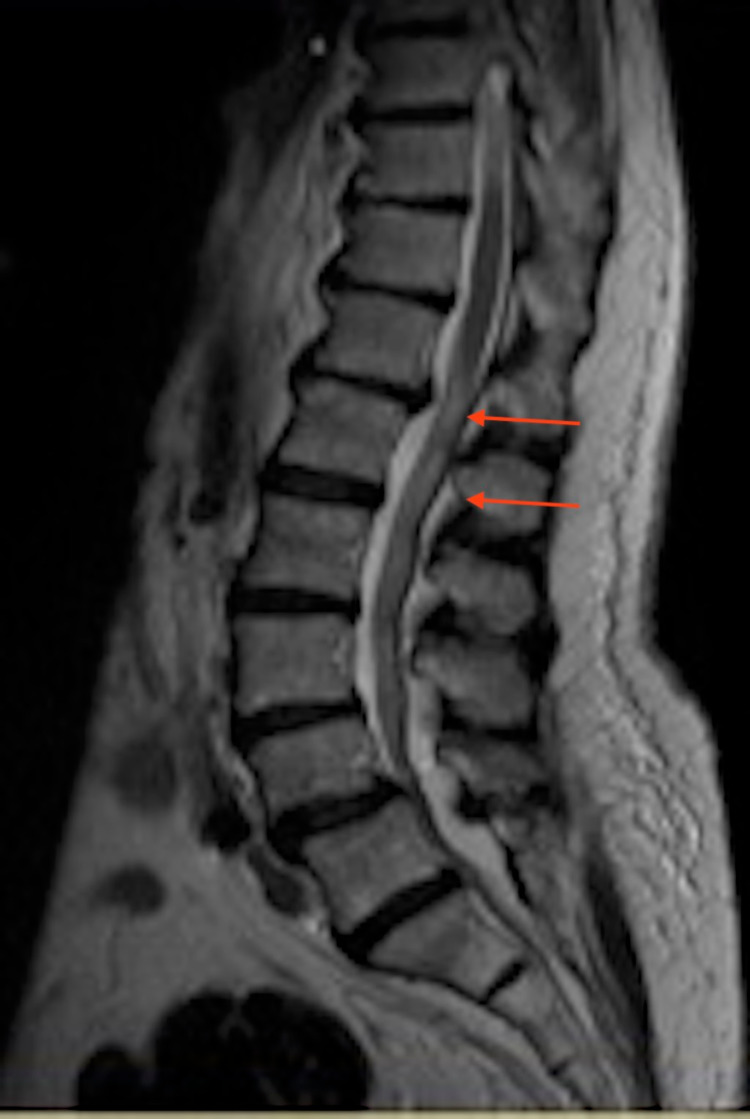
MRI lumbar spine showing arthritis and degenerative disc disease with epidural lipomatosis causing multilevel neural foraminal and spinal canal stenosis most pronounced at L4-L5 and L5-S1 (red arrows)
